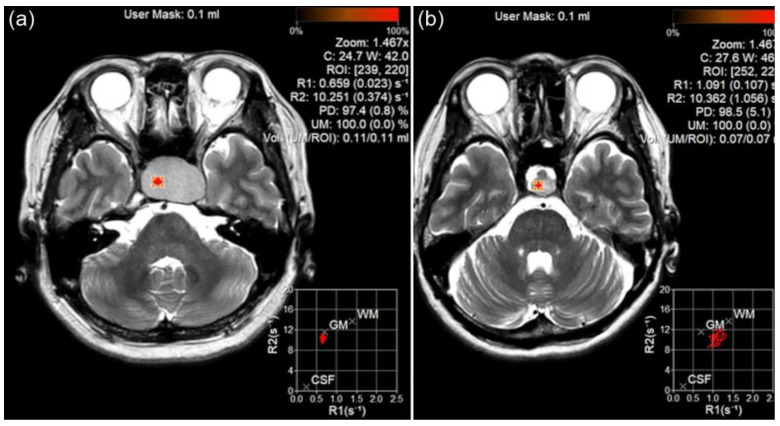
Region of interest (ROI) measurement for pituitary adenoma (PA) and Rathke’s cleft cyst (RCC) using magnetic resonance imaging compilation (MAGiC) image. The ROI was placed on PA (a) and RCC (b), and T1 and T2 relaxation rates were calculated.
